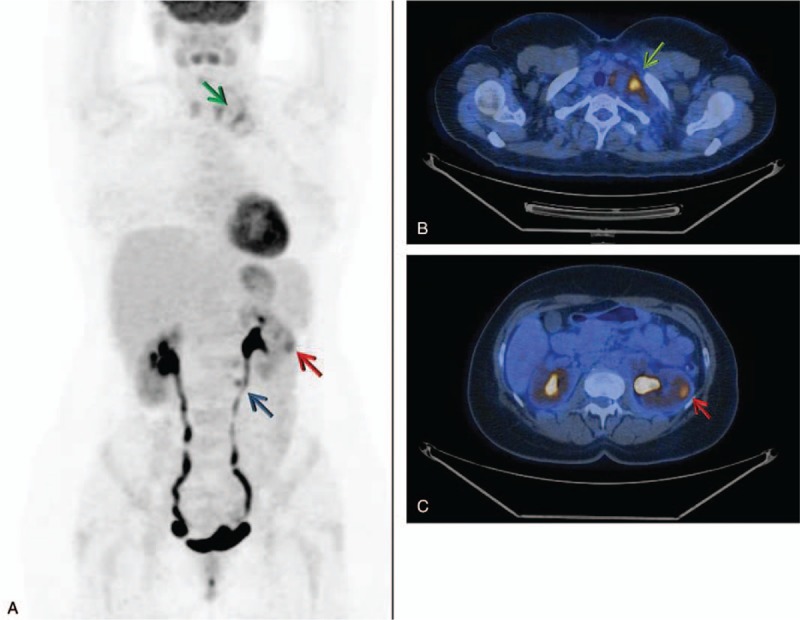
18F-FDG PET/CT (A) MIP and (B-C) axial fusion images showed increased glucose metabolism in the left kidney (red arrows) and in several homolateral lymphnodes in cervical, supra-clavicular (green arrows), and para-aortic sites (blue arrow).
